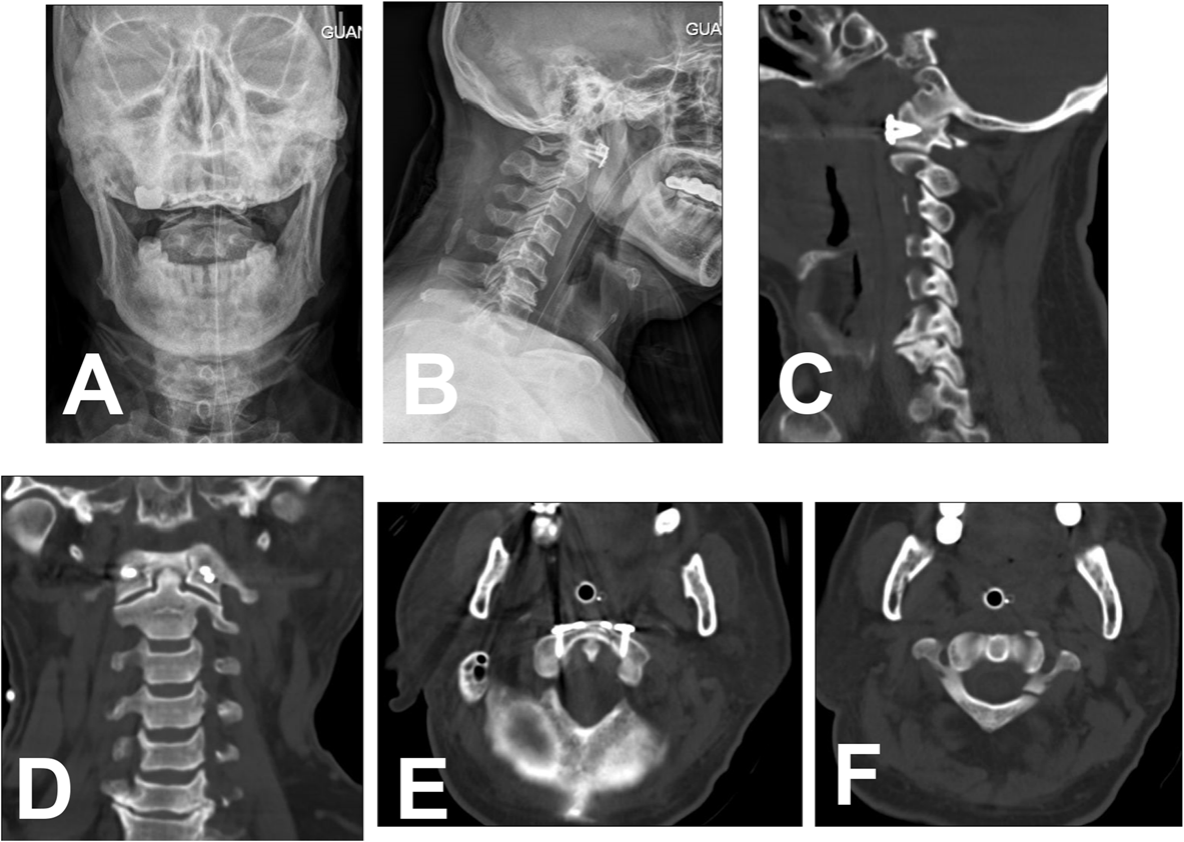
Postoperative X-ray and CT showed a good position of the JeRP plate (A, B, C, D, and E). CT scan showed that the preoperative LMD decreased from 6.54 mm to 0 mm after the surgery (D), and C1 fracture was anatomically reduced (E and F)
